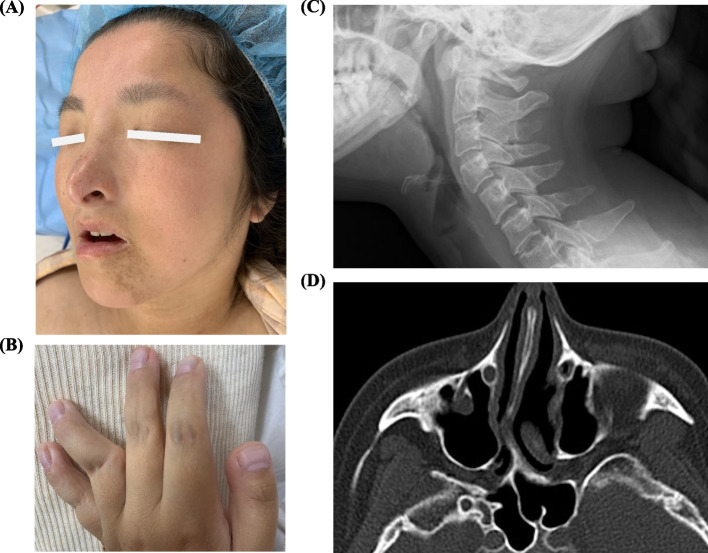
Surface appearance and radiographic images of the patient with Rubinstein-Taybi syndrome. A Slanting, widely spaced eyes and a beak-shaped nose but not remarkable micrognathia. B A broad thumb and a bended ring finger in the left hand. C Non-choanal atresia and airway narrowing in cervical X-ray. D Remarkably deviated nasal septum in brain CT
